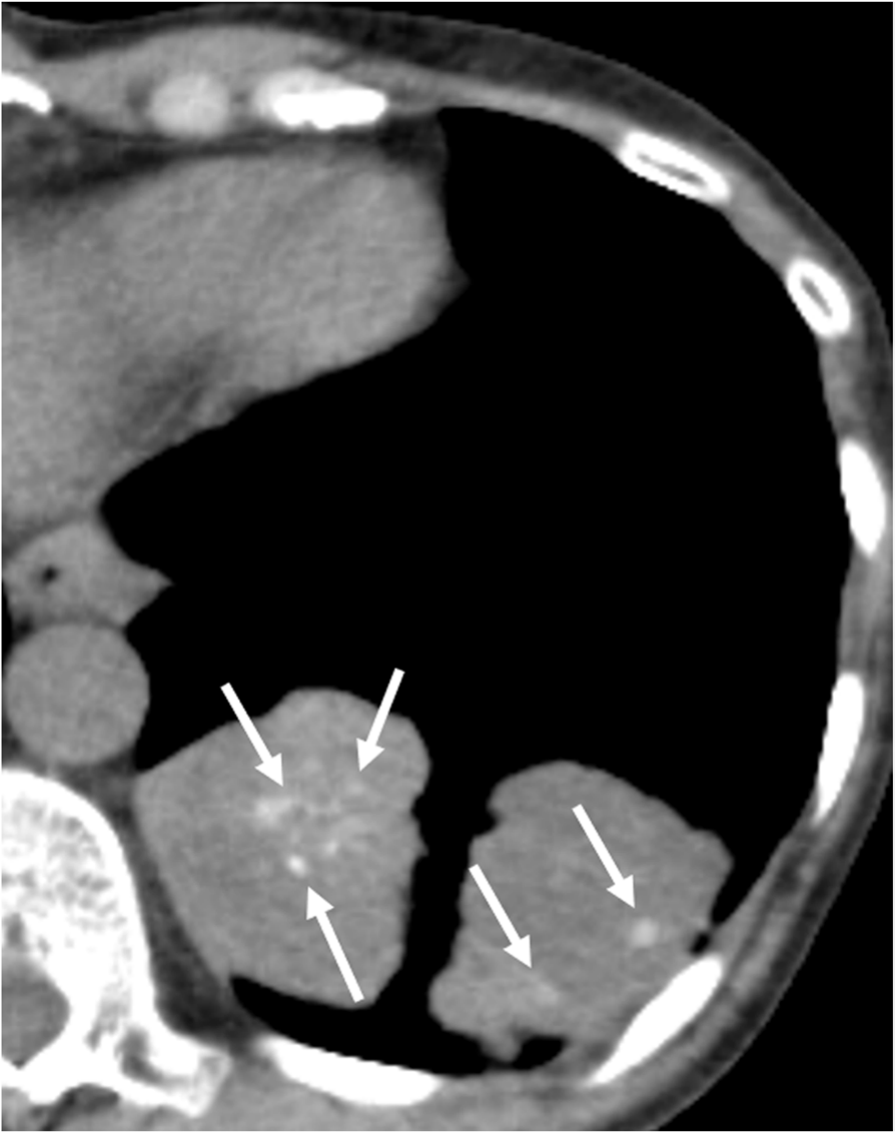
Metastatic colorectal cancer in a 61-year-old woman. CT image shows soft tissue masses with multiple punctate calcifications in the left lower lobe (arrows)
